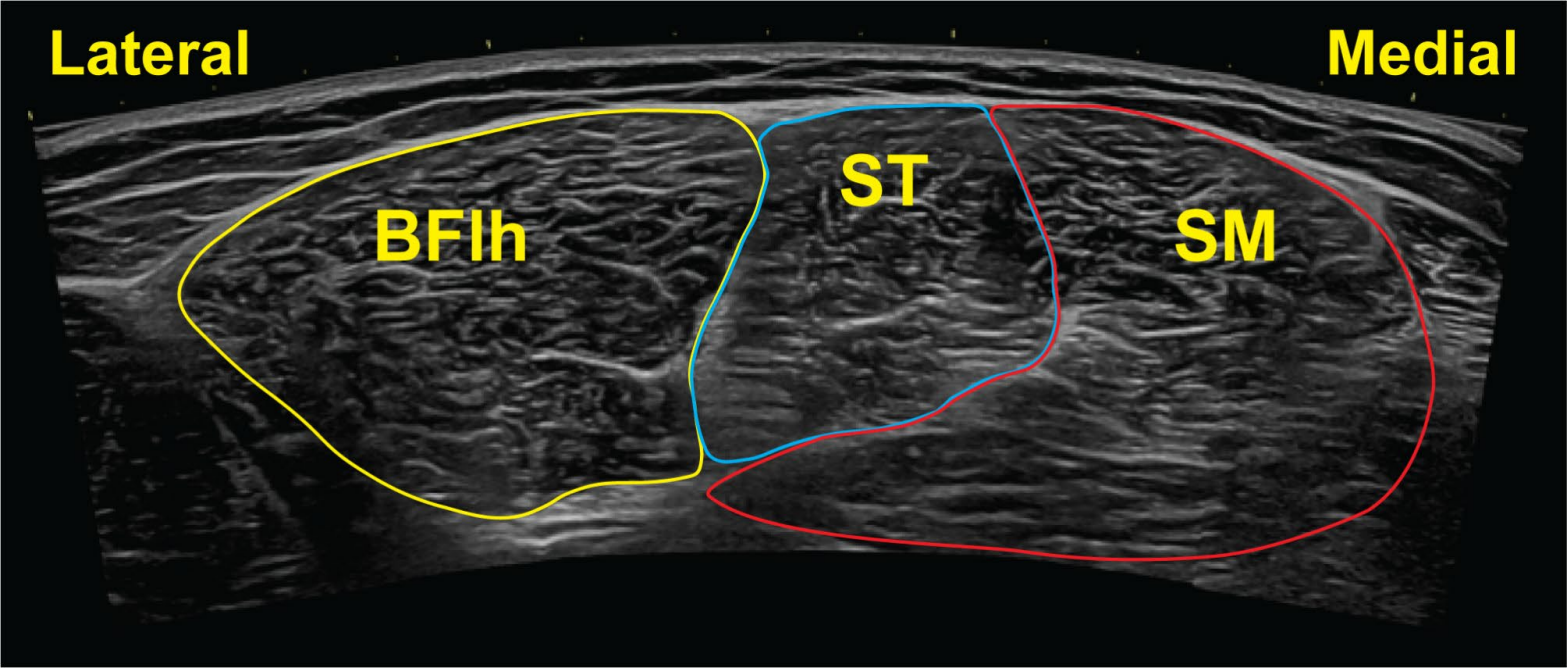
Representative transverse extended-field-of-view image of the biceps femoris long head muscle (BFlh), semitendinosus (ST), and semimembranosus (SM) muscles. Anatomical cross-sectional area will be calculated from the transverse ultrasound images
